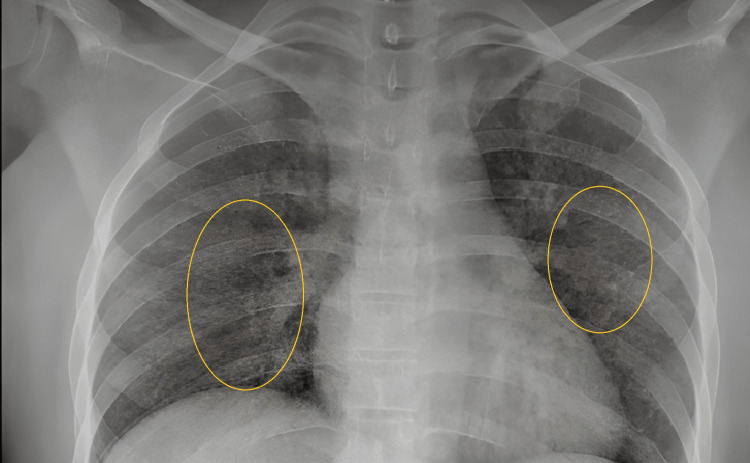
Chest x-ray – miliary mottling of both lung fields
